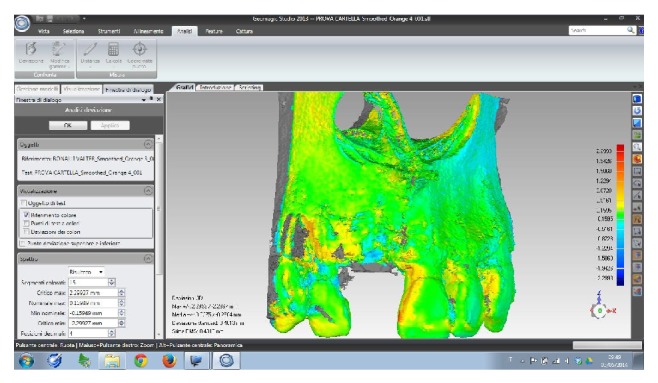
Overlapping of digital images. The DICOM (digital imaging and communication in medicine) files of the obtained CBCT datasets, 6 months and 2 years after grafting with synthetic, micromacroporous biphasic calcium-phosphate, were converted into a surface mesh model with digital imaging software (Mimics, Materialise, Leuven, Belgium). The two surface mesh models were then superimposed and rigidly aligned with anatomical landmarks, with the aid of a software for the overlapping of digital images (Geomagic Studio, Morrisville, NC, USA). The distance between the 2 surface meshes was presented as color-coded graded figures (blue: tissue loss; orange/red: tissue apposition; green/yellow: little or no modifications) to identify zones of bone resorption. In the frontal view, little or no buccal bone loss was evidenced, confirming the stability of the regenerated bone along time.
